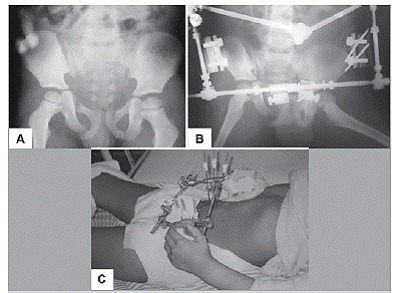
(A) Patient admitted with pelvic ring fracture (Torode and Zieg Type IV). (B) Patient was submitted to stabilization and external fixation of the hip, besides exploratory laparotomy and colostomy, x-rays and clinical image (C).
